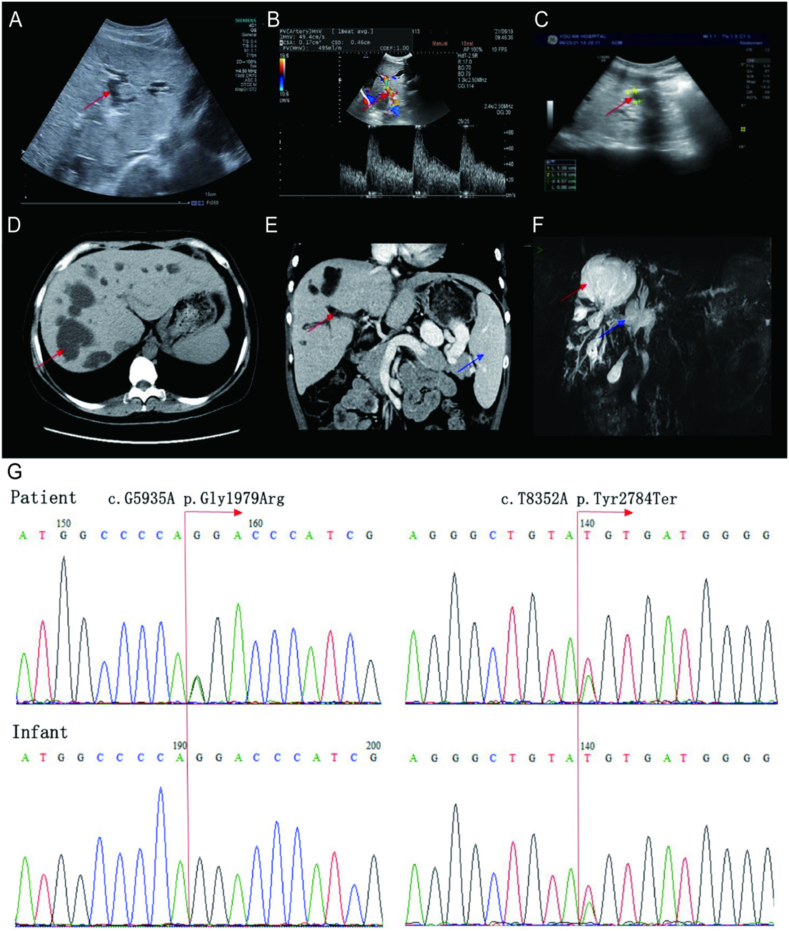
Findings of the imaging examinations. Ultrasonography showed (A) cystic dilatation of the intrahepatic bile ducts (red arrow), (B) occlusion of the main portal vein and its branches (red arrow), and (C) a renal cyst (red arrow). Contrast-enhanced computed tomography revealed (D) a hepatic cyst (red arrow) and (E) splenomegaly (blue arrow). Magnetic resonance cholangiopancreatography revealed (F) cystic dilatation of the intrahepatic bile ducts (red arrow) and a hepatic cyst (blue arrow). (G) Sanger sequencing revealed two mutations in *PKHD1*, c.G5935A(p.Gly1979Arg) and c.T8352A(p.Tyr2784Ter).
